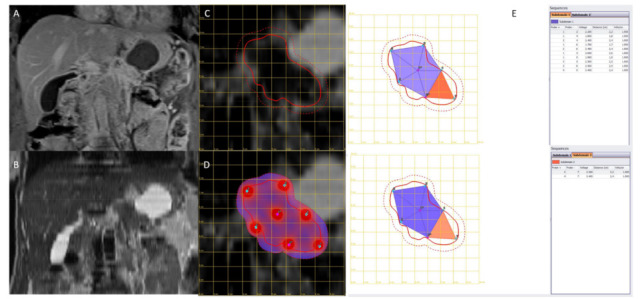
The panel reports an example of preoperative planning performed with the software Pulsar. In (A) (VIBE T1- weighted image post-contrast medium in coronal plane) and (B) (HASTE T2-weighted image in coronal plane) there are the magnetic resonance (MR) sequences used to identify the target areas, (C) represents the definition of the lesion contours, (D) represents the results of the planning in terms of number and position of the electrodes and (E) shows defined electrochemotherapy (ECT) treatment parameters for each subdomain (the treatment was divided into two subdomains, considering that the proposed optimized electrode number to obtain complete coverage of the lesion was 7).
